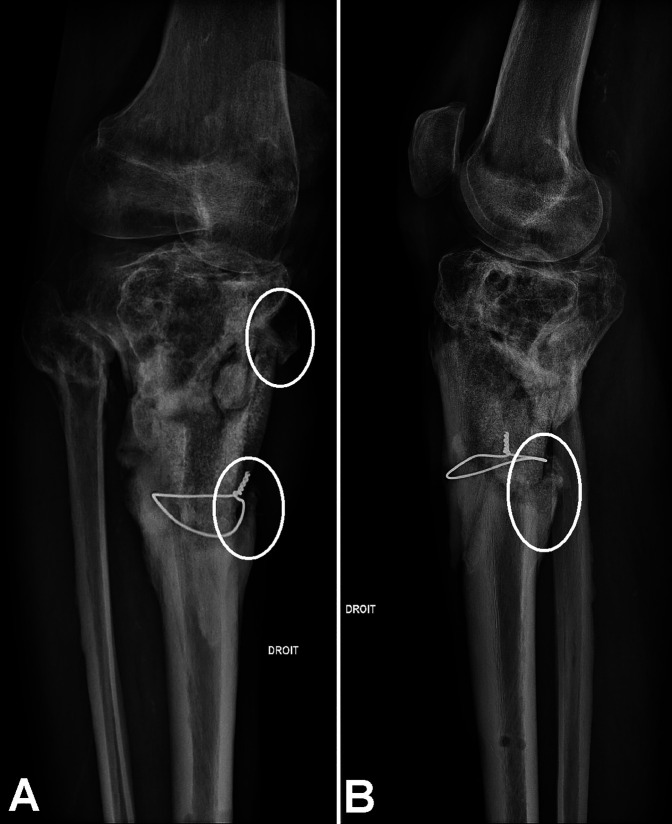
A, Front and (B) side radiograph of the right tibia performed 18 months after ceramic implantation. White circles indicate bone growth.
